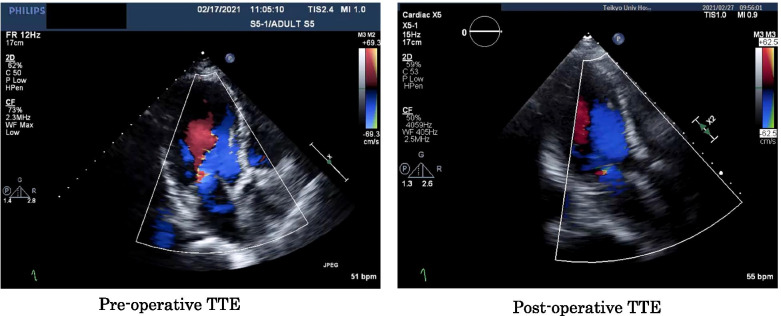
Pre- and postoperative transthoracic echocardiography (TTE). Comparison of post-operative TTE and that of pre-operation. Mitral regurgitation was relieved
